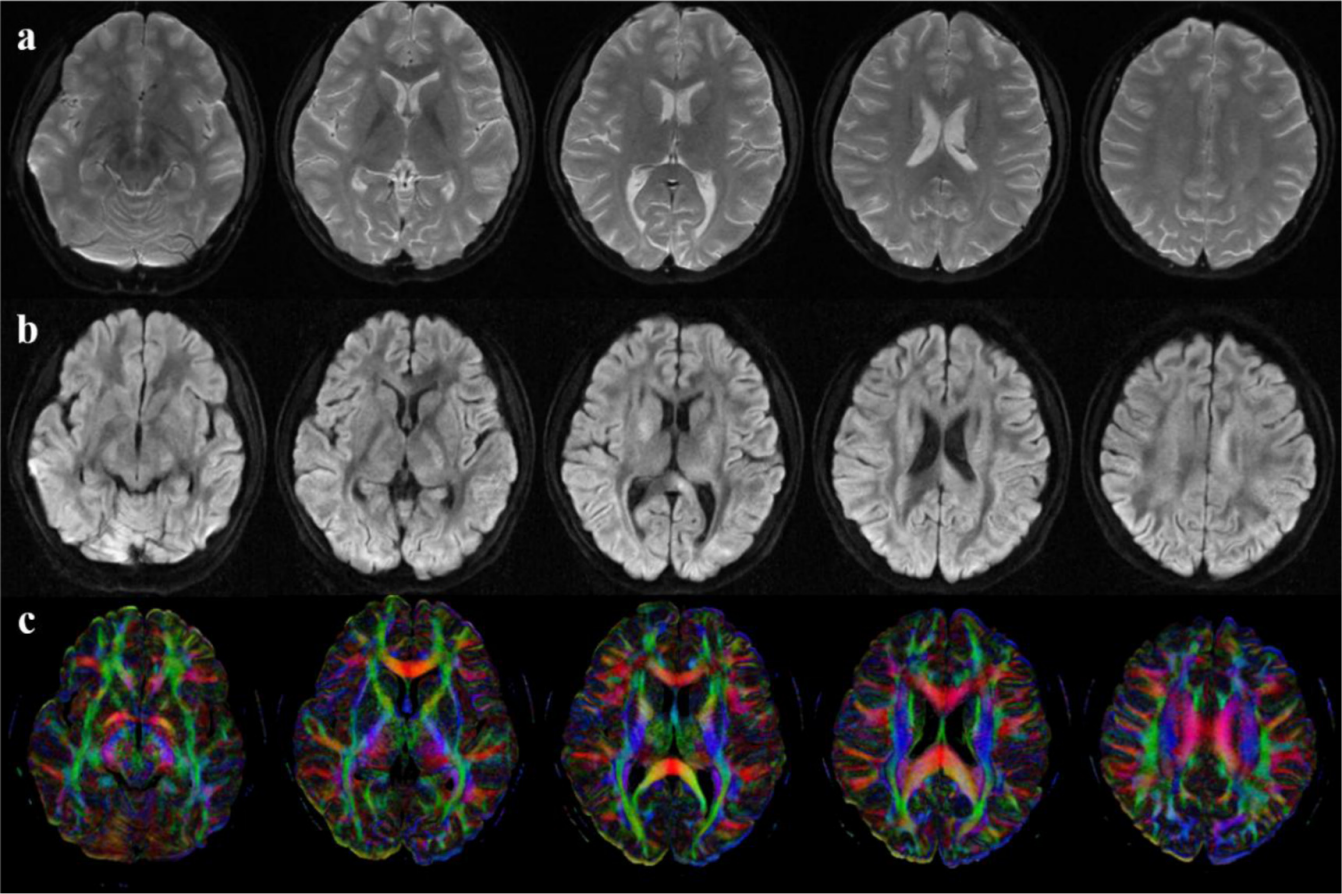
DWI and FA from a representative subject. a) b = 0 images; b) DWI images; c) FA maps. Five representative slices are shown from the left to the right.
